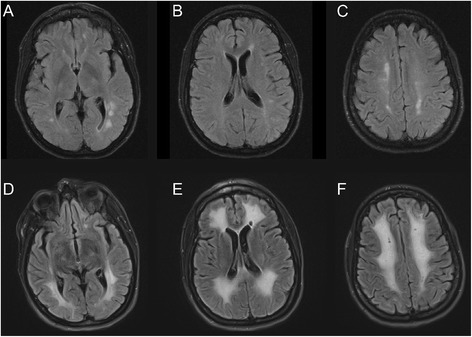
Case 2: MR images (FLAIR) of brain lesions. a-c On admission foci of increased signal are present in the white matter of the cerebral hemispheres bilaterally, predominantly in a periventricular distribution. d-f Repeat imaging seven weeks later, after further neurological deterioration, shows prominent interval progression of signal abnormality in the subcortical and periventricular white matter
